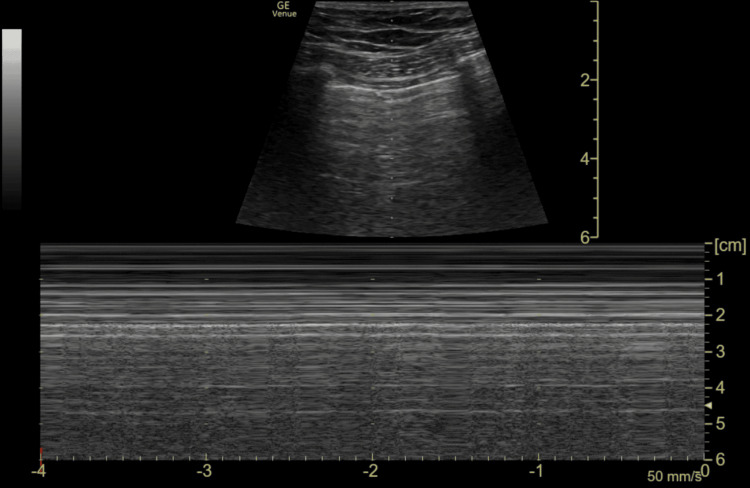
Lung ultrasound of the right lung. B-mode and M-mode images demonstrate normal lung sliding with a seashore sign, excluding pneumothorax
